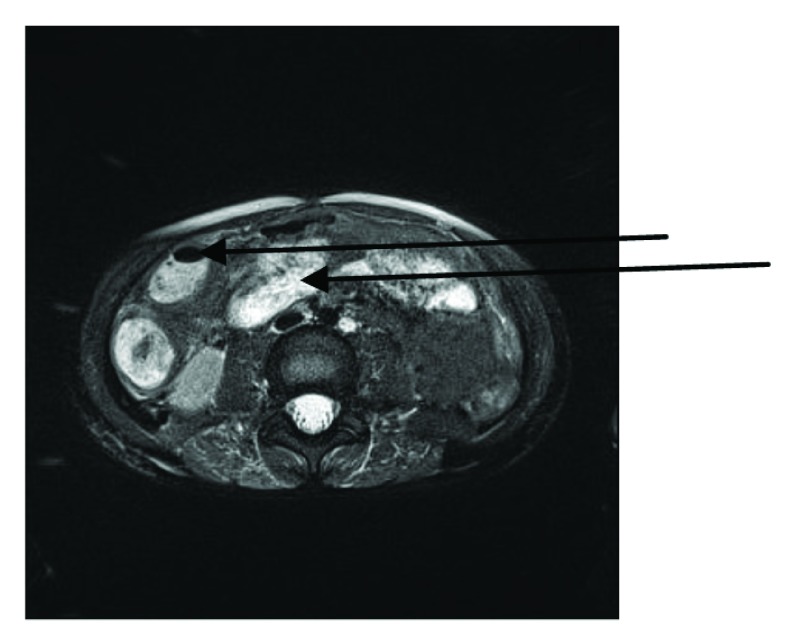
MRI scan of abdomen obtained on the 12th day of disease: edematous jejunal wall and distension with air fluid level in caecum (arrows).
